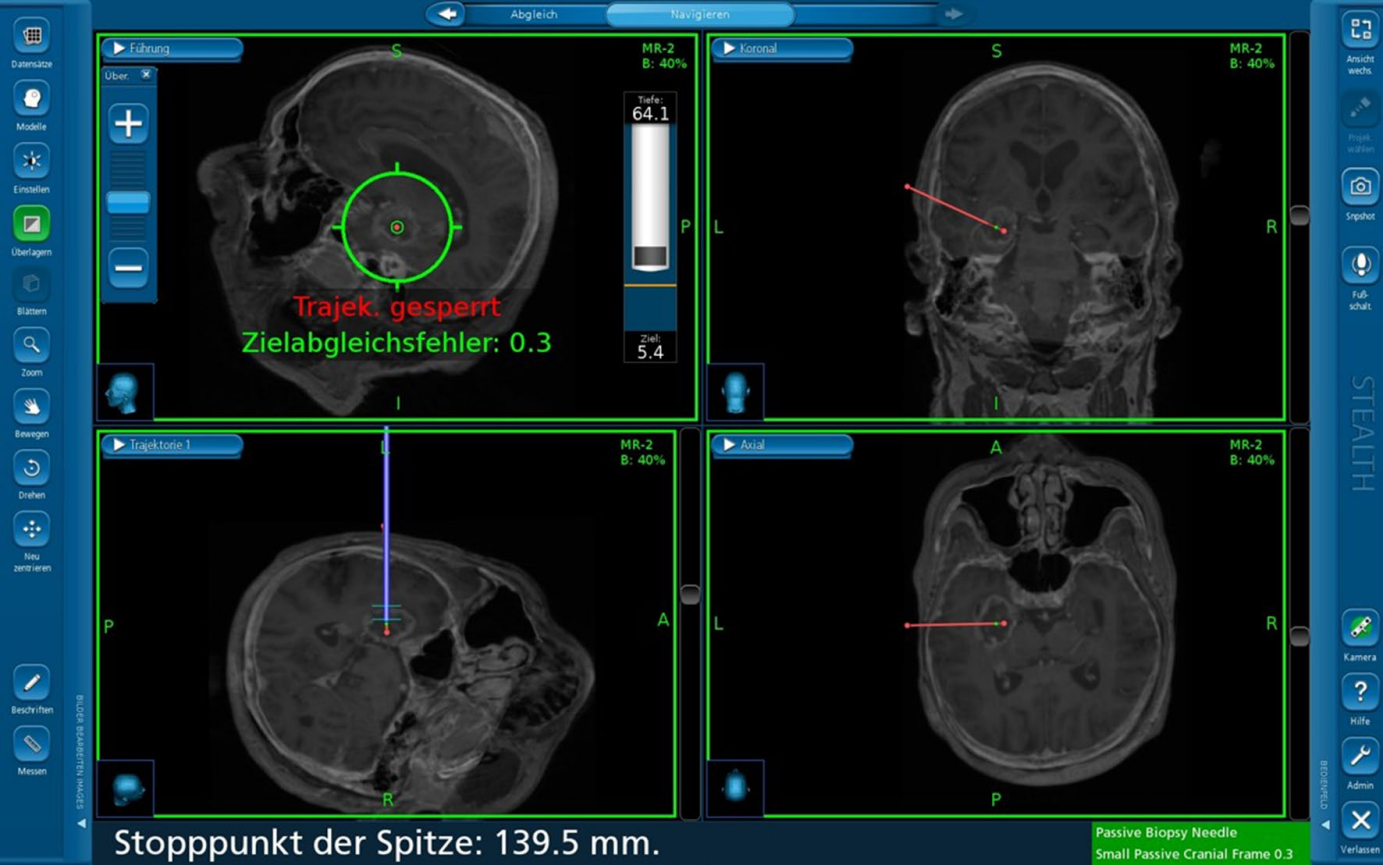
Trajectory planning and stereotactic biopsy under 50% overlay of the MRI image data set
